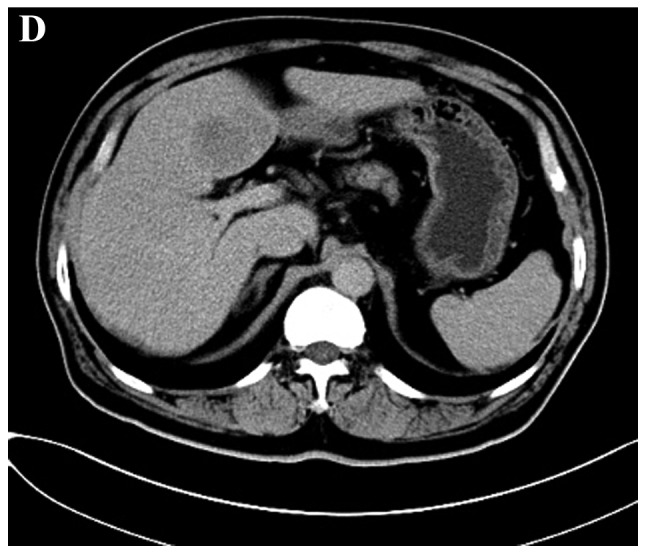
CT images of the lesion. (A) On the plain CT scan, an oval homogeneous hypodense mass with a distinct border is located in the quadrate lobe of the liver. (B) Arterial, (C) portal venous and (D) delayed phase CT scan showing slight and continued enhancement in the peripheral of the mass. CT, computed tomography.
